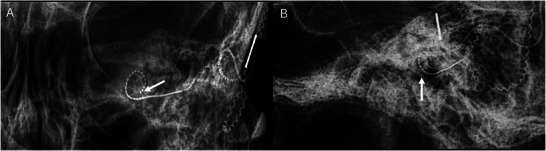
Radiographs of the temporal bone and inner ear following cochlear implantation. Radiographs in the anteroposterior (A) and lateral (B) views demonstrate the electrode array positioned within the cochlea (arrow).
